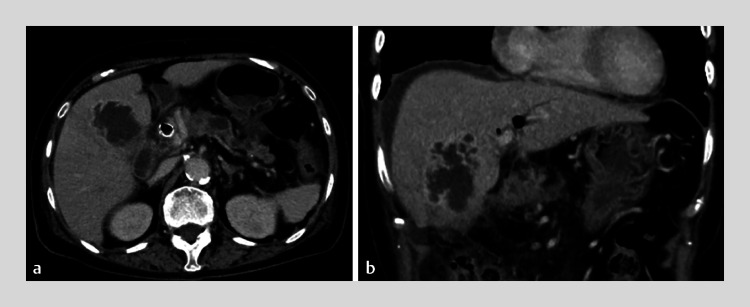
Contrast-enhanced computed tomography image showing:
